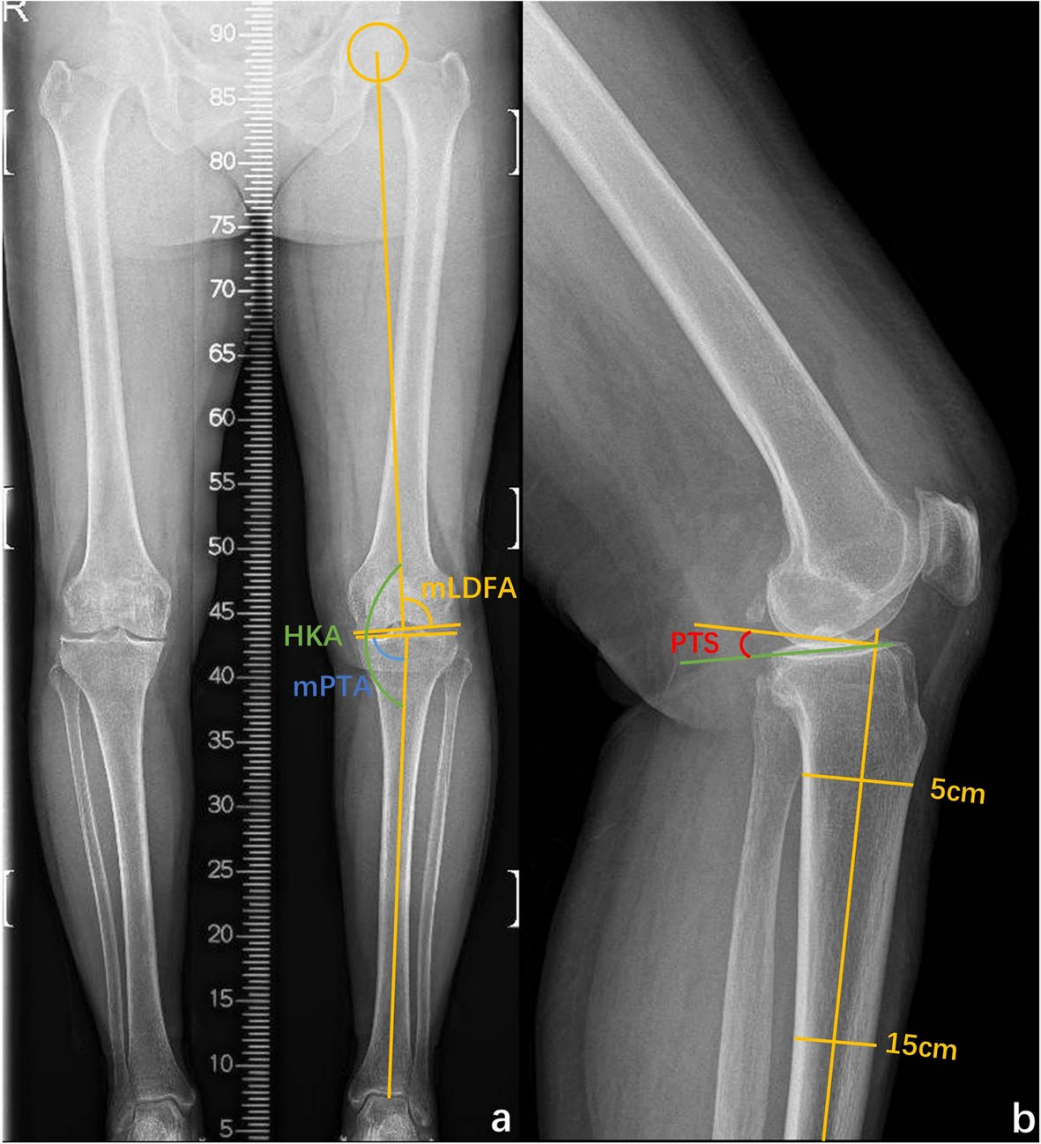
Measurement of different alignment parameters on hip-to-ankle anterior–posterior (AP) standing (a) and lateral (b) X-rays. Hip–knee–ankle angle (HKA) is the angle between the tibial mechanical axis and femoral mechanical axis, mechanical proximal tibial angle (mPTA) is the angle between the tangent of the medial and lateral tibial plateau and the mechanical axis of the tibia, and mechanical lateral distal femoral angle (mLDFA) is the angle between the tangent of the medial and lateral femoral condyle and the mechanical axis of the femur. Posterior tibial slope (PTS) is the angle between the tibial anatomical axis (a straight line connecting the midpoint of the line at 5 cm and 15 cm from the knee joint line) and tibial plateau (a line connecting the anterior and posterior points of the most proximal part of the tibial plateau)
